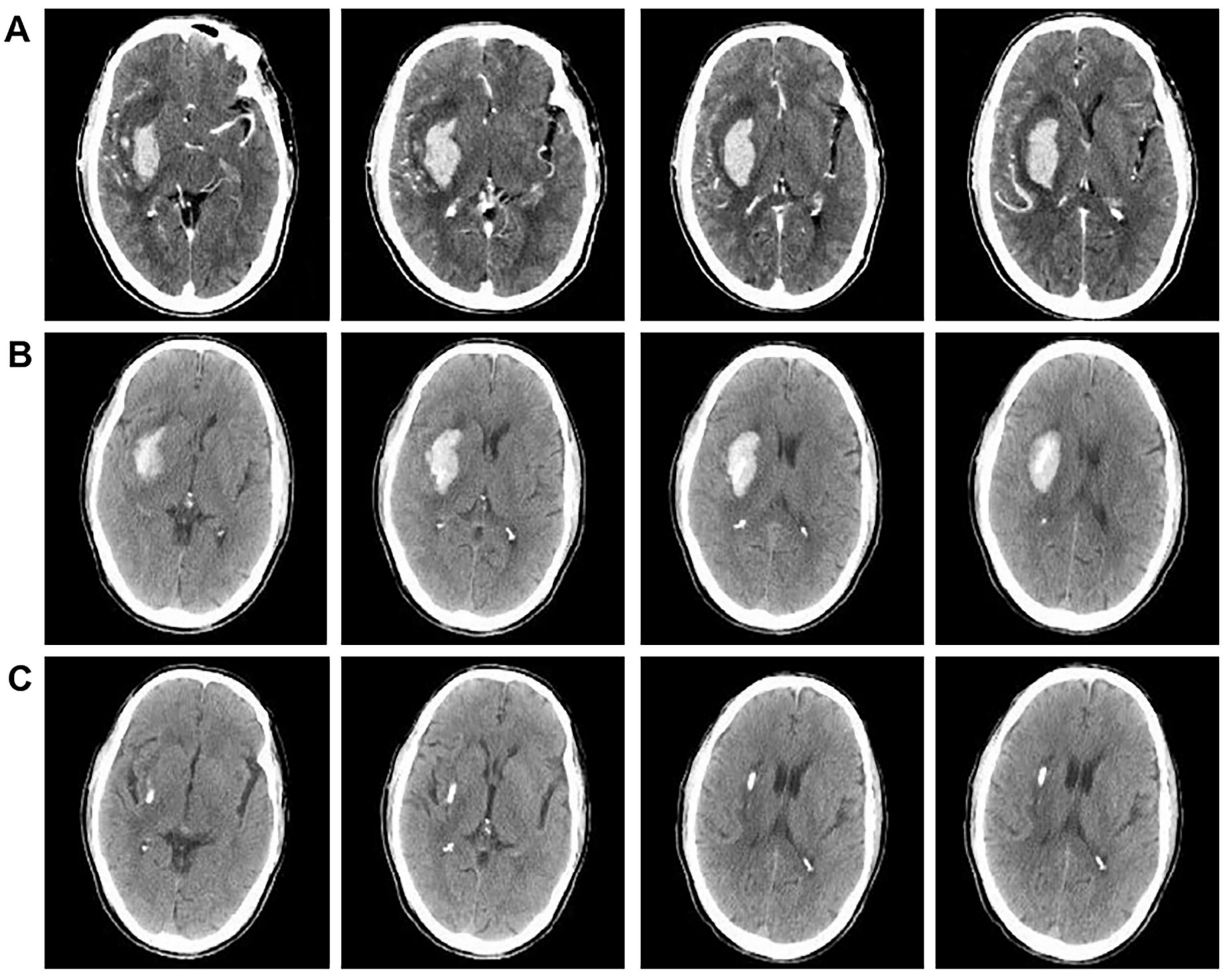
CT comparison images before and after surgical intervention. (A) Brain CTA scan images of patients upon admission. (B) Brain CT scans of patients 24 h post-surgery. (C) Brain CT scans of patients 3 days post-surgery.
